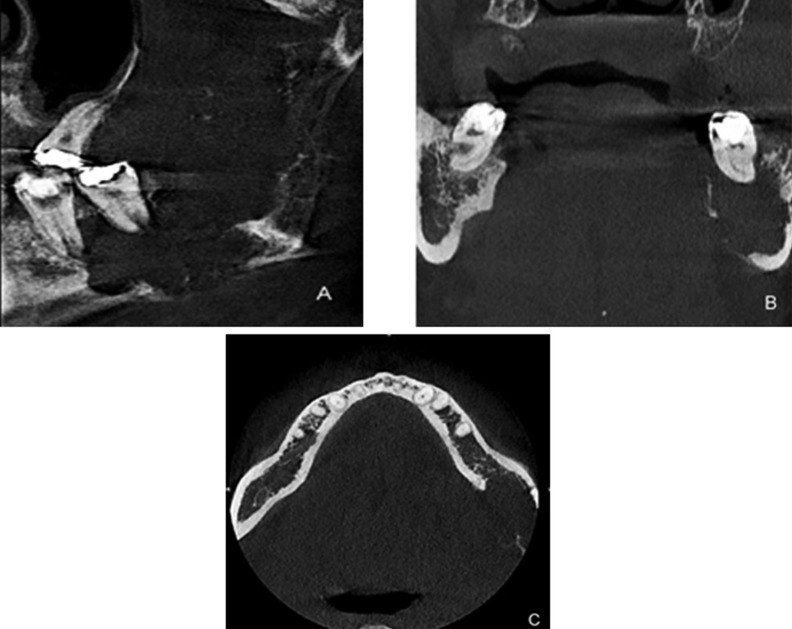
A: Sagittal CBCT image reveals expansile hypodense lesion in the inferior portion of the left ramus with thinning and expansion of the cortical portion; B: Coronal view an expansile lesion involving the left mandible: note the bone destruction; C: Image showing axial view.
